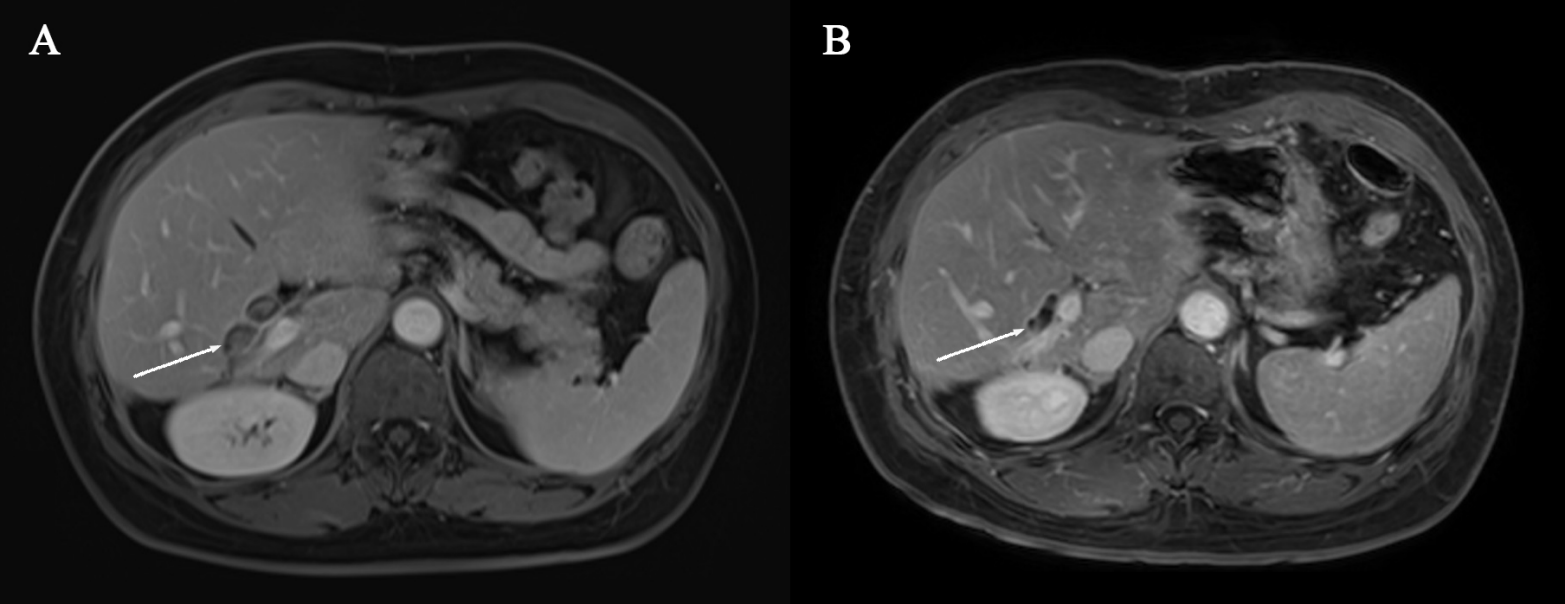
(A) Pre-systemic therapy enhanced-contrast MRI image during portal phase (B) Post-systemic therapy enhanced-contrast MRI image during portal phase. Arrow indicates biliary duct carcinoma thrombosis in (A, B).
